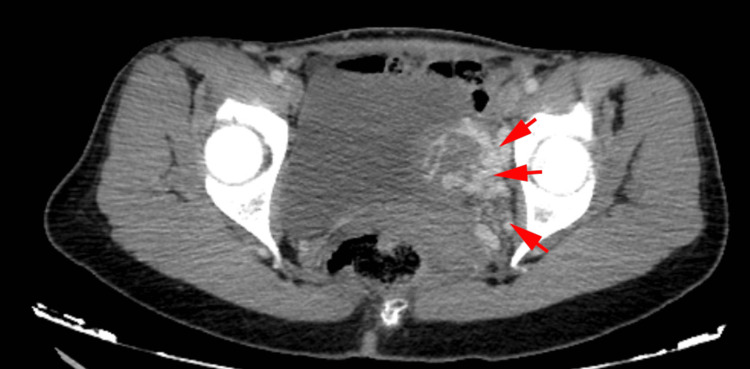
CT scan of the pelvis (axial view) The red arrows are showing pelvic varices.
